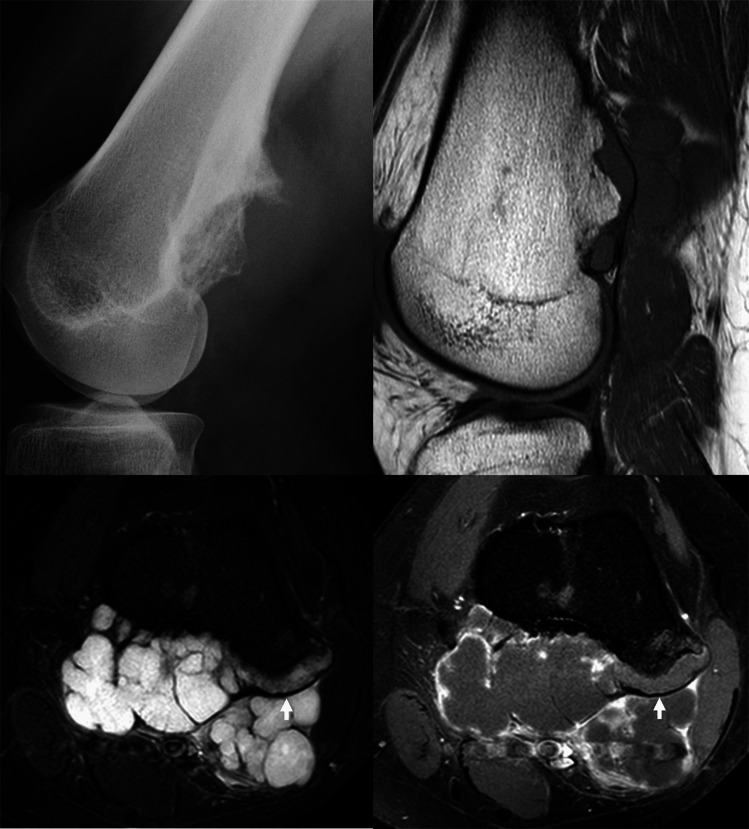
Twenty-six-year-old female with peripheral atypical cartilaginous tumour. Lateral radiograph (top left) demonstrates a surface lesion with regions of mature ossification, lucency, cortical erosion and prominent underlying sclerosis. Sagittal T1-weighted image (top right) demonstrates mature ossification corresponding to the residual osteochondroma with a non-thickened low signal cartilage cap, in addition to an overlying lobular mass showing similar signal. Axial PD (bottom left) and T1FS post-contrast (bottom right) images reveal lobular chondral signal tissue with peripheral, septal and nodular post-contrast enhancement which extends medial and superficial to the residual osteochondroma and non-thickened chondral cap (arrow)
